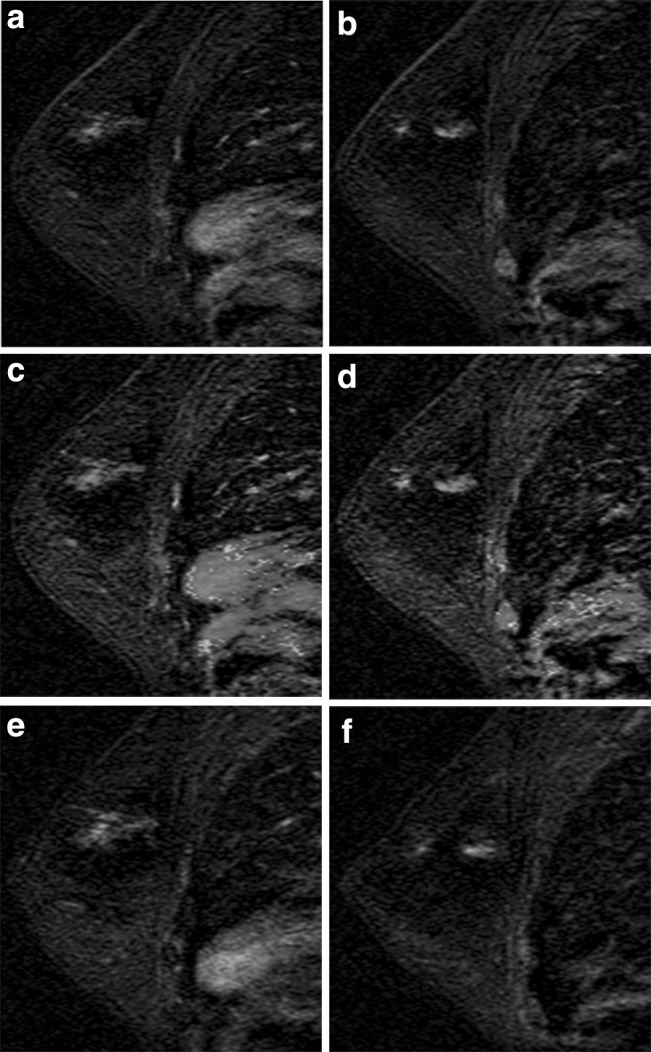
Multiple regions of non-mass enhancement (NME) in the same breast. Postcontrast subtraction T1-weighted images a, b show multiple regions of NME, which are new but demonstrated persistent kinetics (c, d). These were assessed as probably benign, given the multiplicity, and were assumed to be transient enhancement related to hormonal status in this premenopausal woman. Follow-up exam 6 months later demonstrates slight increase in degree of enhancement (e, f); therefore, MRI-guided biopsy was recommended and yielded ductal carcinoma in situ (DCIS). Patient elected mastectomy yielding diffuse DCIS, no invasive component. New areas of NME should raise suspicion and biopsy should be considered
